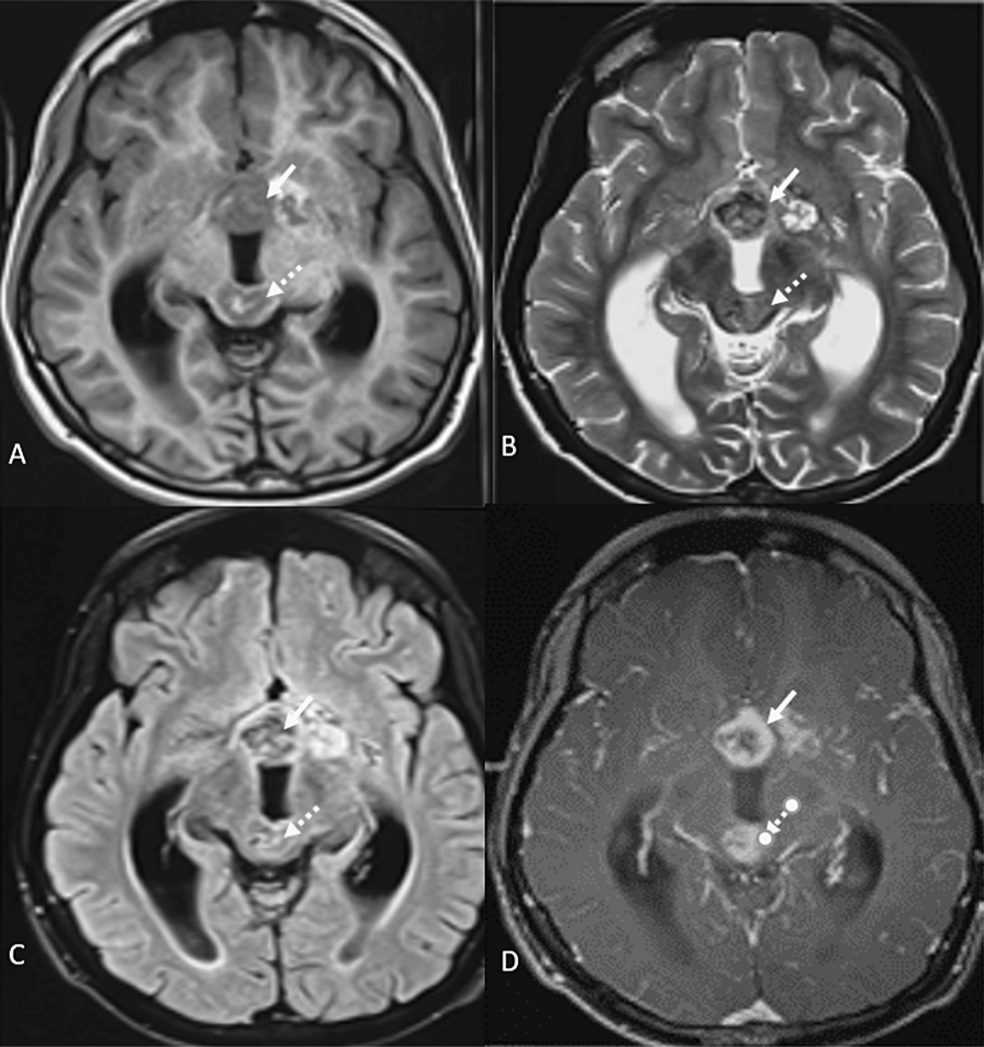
(A) MRI of the brain axial-T1 weighted image showed that the lesion involving the sella, suprasellar (bold arrow) and parasellar regions, and pineal gland (dotted arrow) was heterogeneously hypointense on T1-weighted images with few hyperintense areas within. (B and C) MRI of the brain axial T2-weighted and fluid-attenuated inversion recovery (FLAIR) images, respectively, showed a heterogeneously hypointense lesion involving the sella, suprasellar (bold arrow), and parasellar locations, and pineal gland (dotted arrow). (D) MRI of the brain axial post-contrast image showed that the suprasellar (bold arrow) and pineal gland (dotted arrow) lesions showed heterogenous enhancement on the post-contrast image with central non-enhancing areas.
